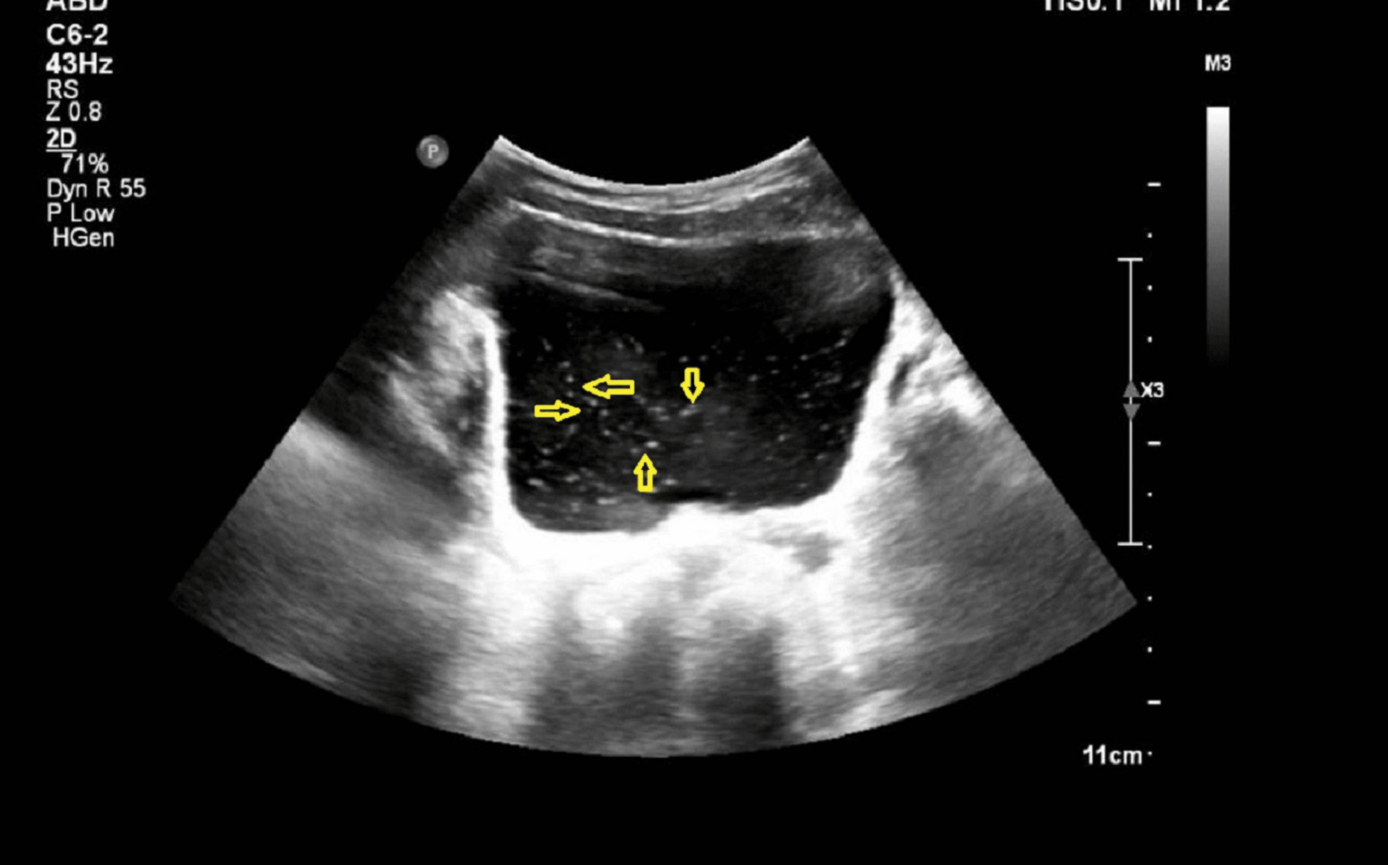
Ultrasound of the kidneys, ureters, and bladder (KUB) revealed floating intraluminal echoes within the urinary bladder (yellow arrows).
